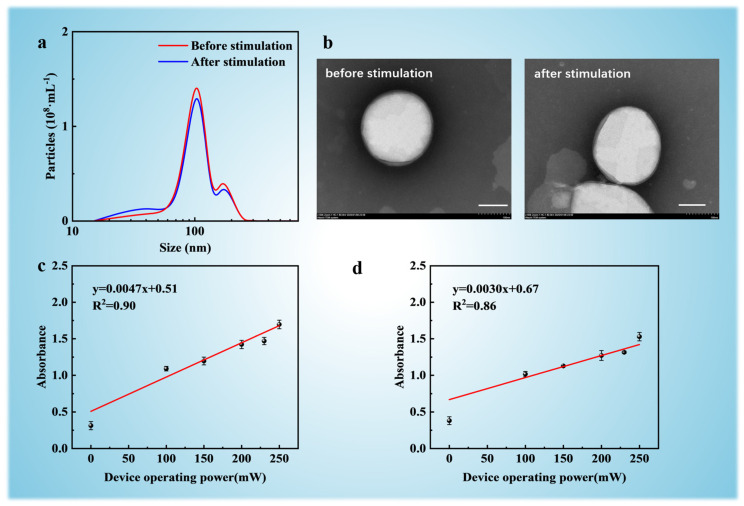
The experimental results of DOX loading into SUVs. (a) Particle size distribution measured by DLS before and after the stimulation of acoustic streaming (250 mW, 10 min). (b) TEM images of SUVs before and after acoustic streaming stimulation (250 mW, 10 min). Scale bars indicate 100 nm. Absorbance of DOX-loaded SUVs under acoustic streaming at different input power levels (0, 100, 150, 200, 230, and 250 mW) based on two methods. (c) DOX is first delivered to GUVs, which are then downsized into SUVs; (d) SUVs are prepared first, followed by direct DOX loading.
